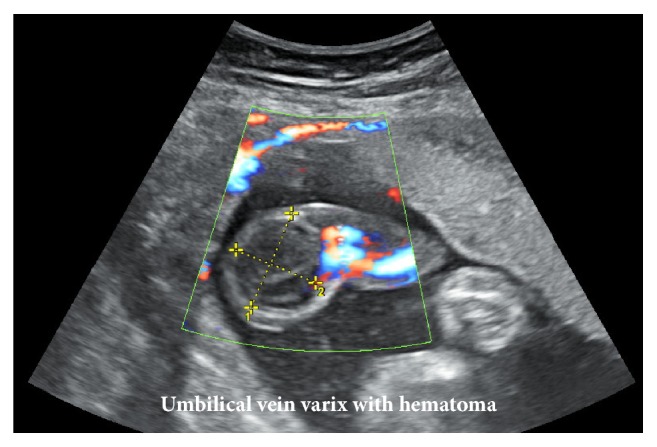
Ultrasonogram at 28 weeks of gestation shows enlargement of the thrombus or hematoma (measured 29 × 24 mm) beside the umbilical vein varix (UVV).
